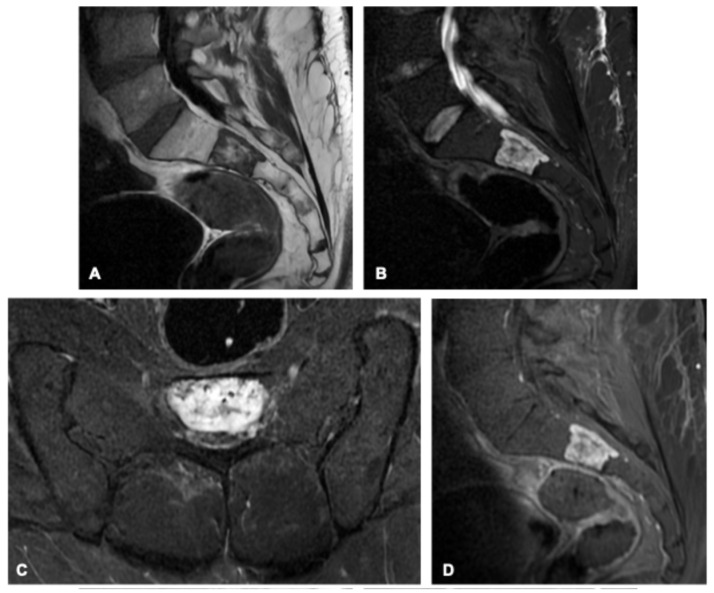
Intraosseous Hemangioma in a 44-year-old man. (A) Sagittal T1-weighted image shows a well-defined, predominantly T1-hyperintense intraosseous lesion in the S2 vertebral body, consistent with fatty stroma. (B) Sagittal T2 fat-suppressed image demonstrates marked hyperintensity. (C) Axial T2 fat-suppressed image best depicts thickened internal trabeculae, producing the characteristic punctate (“polka-dot”) pattern. (D) Post-contrast sagittal T1 fat-suppressed image shows enhancement of the lesion, without an extraosseous soft-tissue component.
